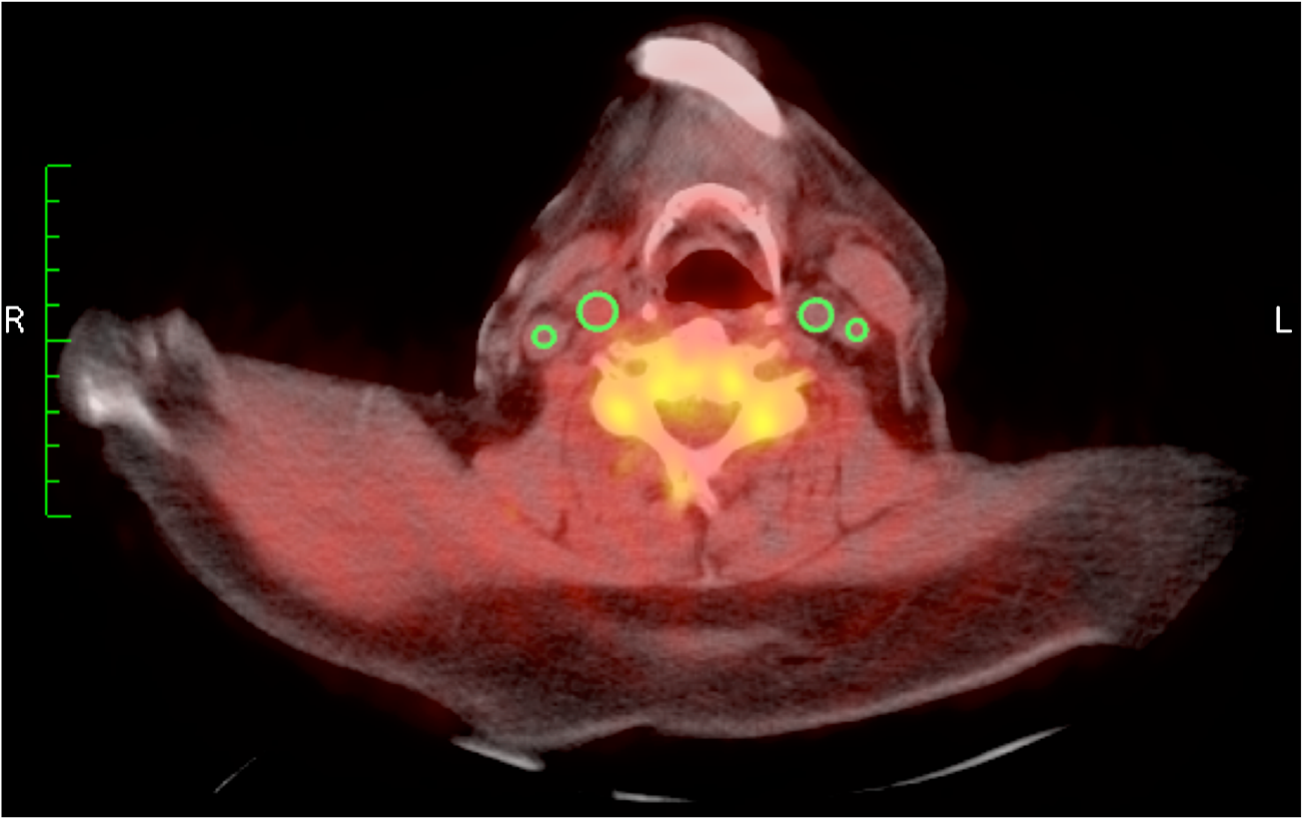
α4β2-nAChR PET/CT scan showing [^18^F]-2-fluoro-A85380 uptake in the right and left common carotid arteries (upper ROIs *green*) and in the right and left jugular veins (lower ROIs). On a visual basis, the fused PET/CT image shows higher uptake of the tracer in the two common carotids compared with the two veins, indicating a specific tracer uptake in the arteries. Furthermore, the right common carotid artery shows a higher uptake (SUV_max_ 1.7 vs. SUV_max_ 1.2 in the left common carotid artery) compared with the left common carotid artery, whereas the uptake in the two jugular veins is almost identical (right SUV_mean_ 1.0 vs. left SUV_mean_ 1.1), further indicating a specific arterial uptake of the PET tracer as well as a higher density of the α4β2-nAChRs in the right than in the left common carotid artery
